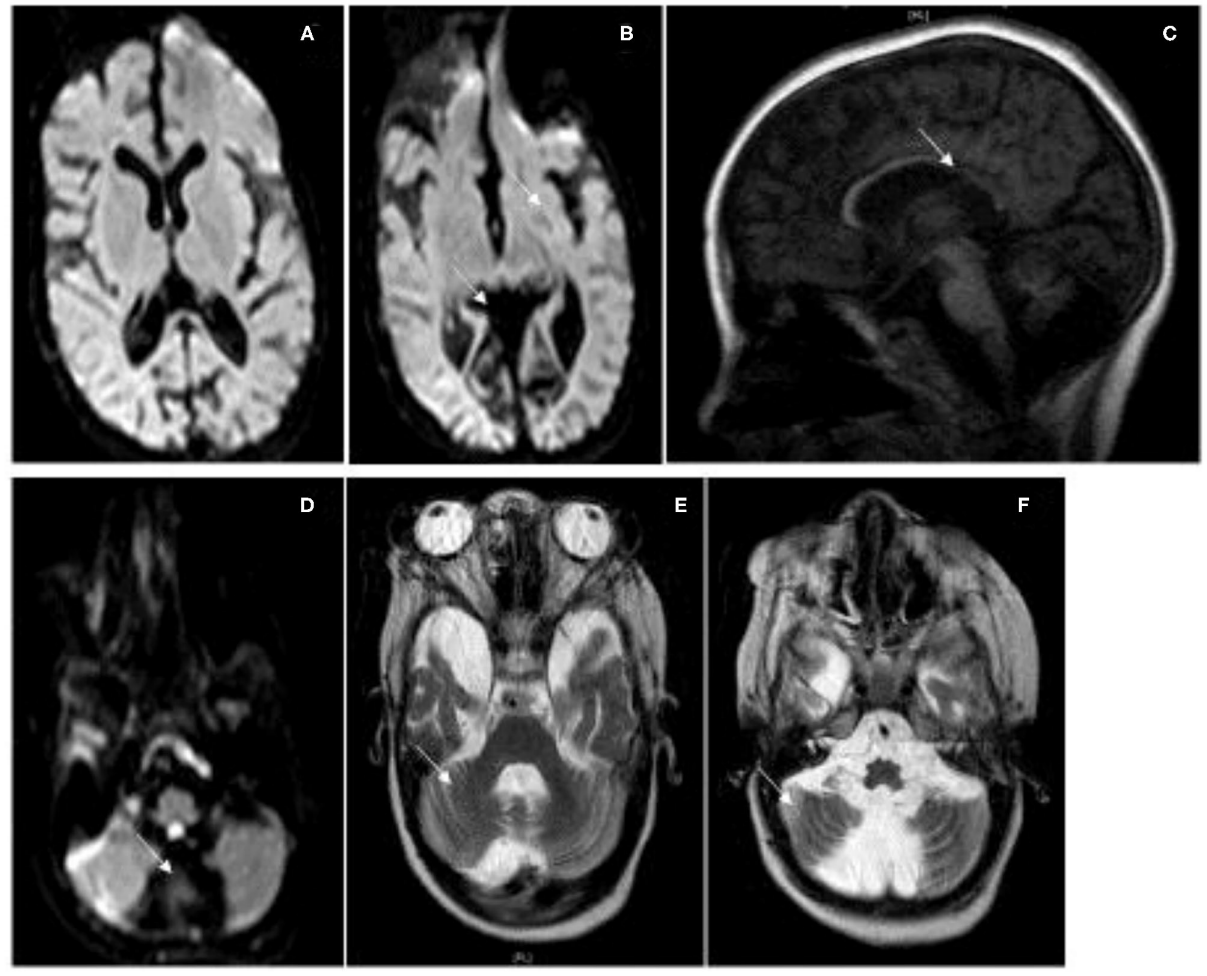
MRI Images. Cranial MRI of patient I at the age of 10 years. (A,B) Flair, (C) T1, (D) Flair, (E,F) T2. MRI shows severe atrophy of the cerebral cortex especially of the occipital cortex and a severe atrophy of the cerebellar hemispheres with a loss of the cerebellar vermis, a thin corpus callosum, and optical atrophy.
